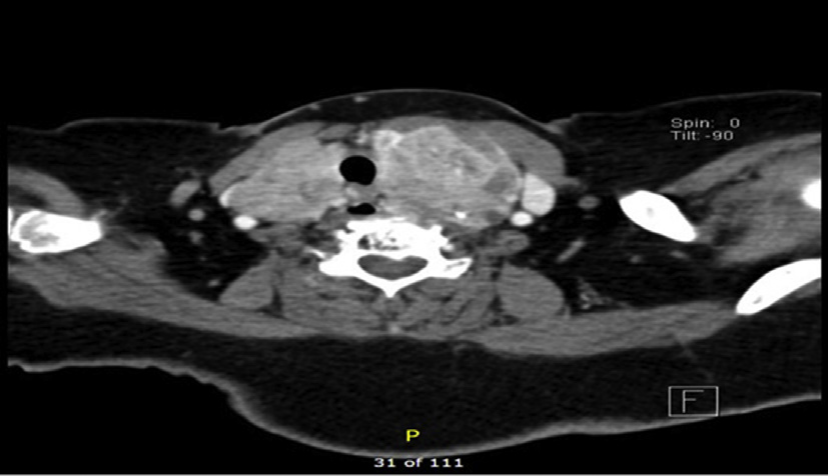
CT with contrast, Axial view demonstrating heterogeneous enlarged left thyroid lobe shifting the airway to the right side.
